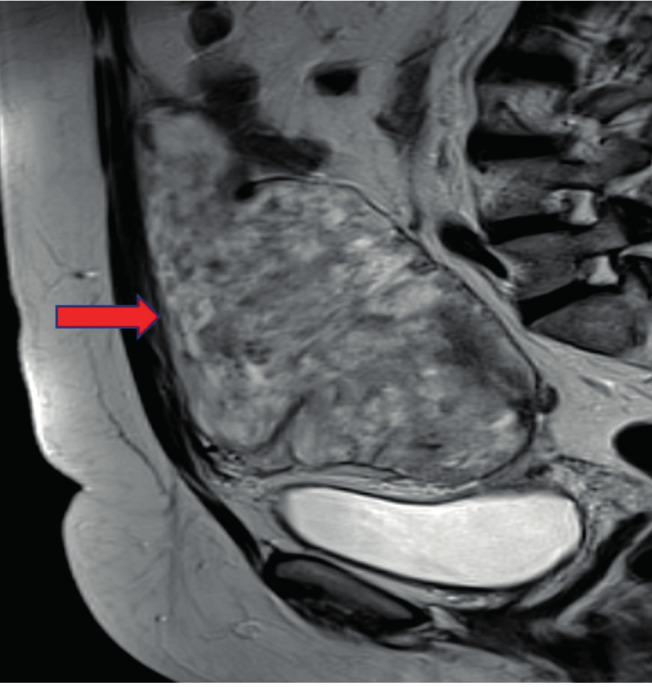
Longitudinal MRI image of the pelvis showing the extensiveness of the parasitic leiomyoma (red arrow: section line between abdominal wall and tumour).
